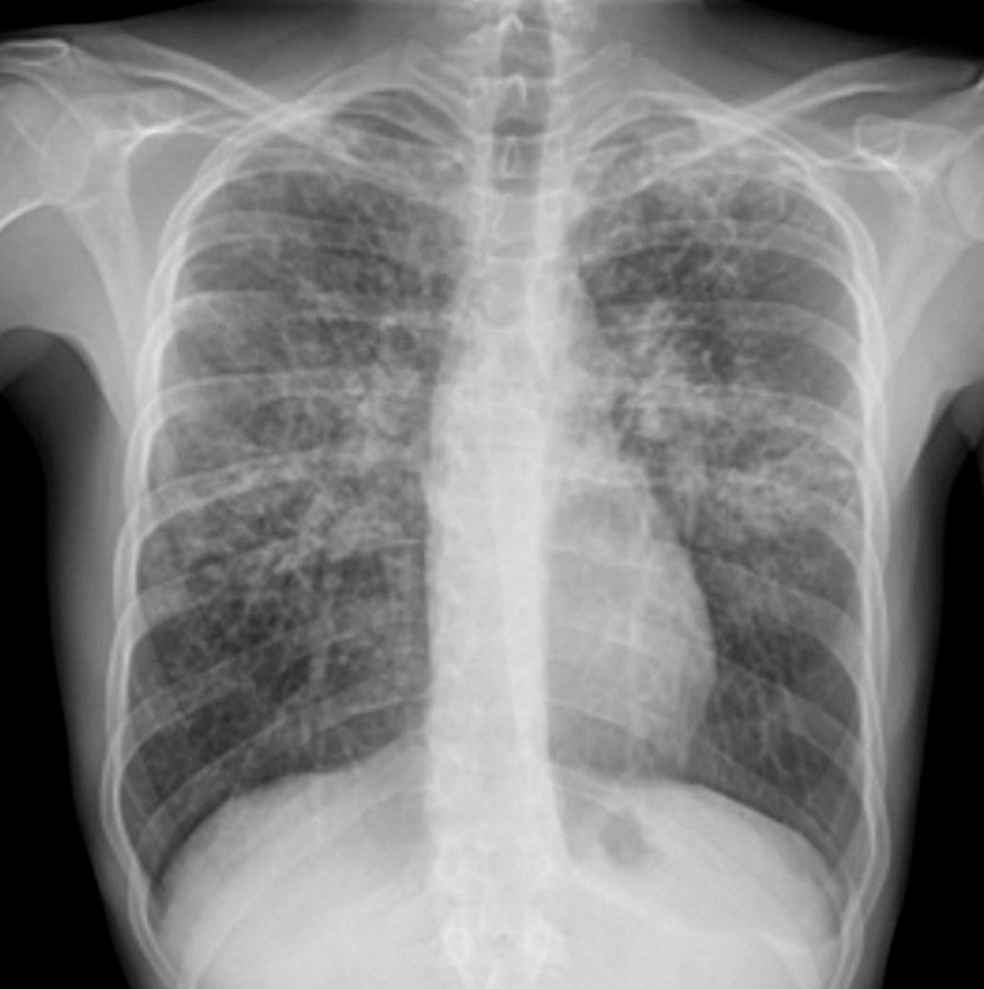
Chest X-Ray nine weeks prior to admission revealing bilateral hilar lymphadenopathy
